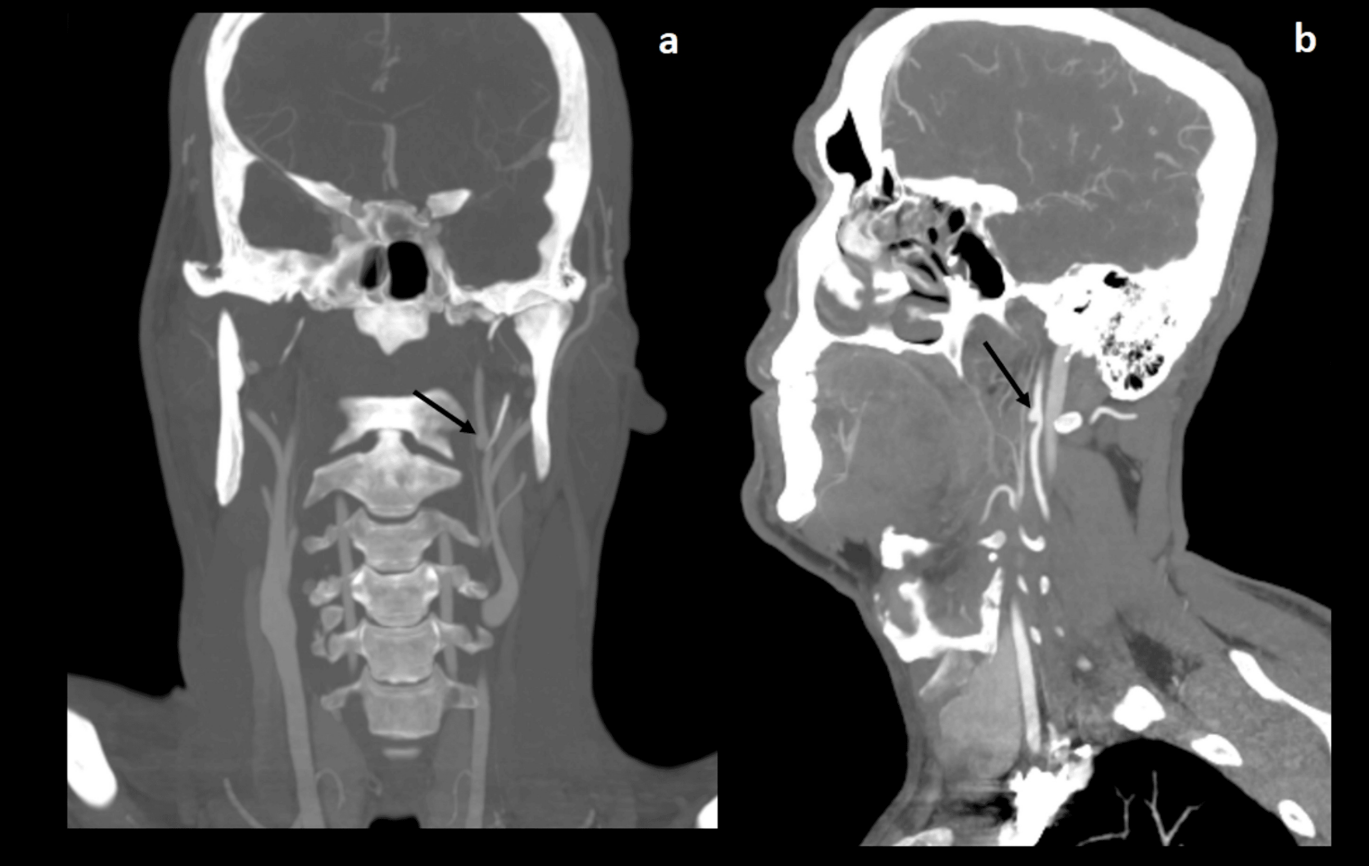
Case 1 coronal (a) and sagittal (b) CT scans demonstrating a pseudoaneurysm of the left ICA (black arrows) CT: computed tomography, ICA: internal carotid artery
